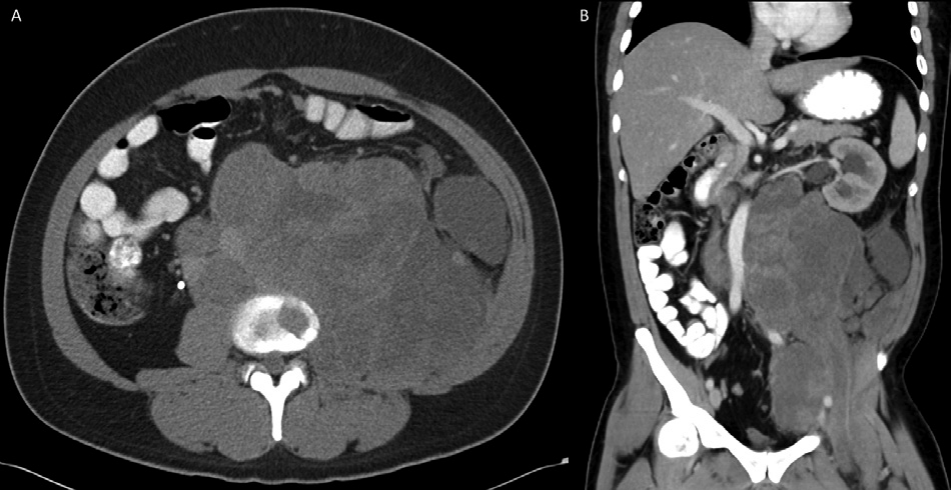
Axial (Fig. 1A) and coronal (Fig. 1B) computed tomography (CT) image of the abdomen 2 years following the initial diagnosis demonstrate a large heterogenous soft tissue mass arising from the left iliopsoas muscle. There was significant mass-effect on the abdominal viscera, with cranial displacement of the left kidney and right-ward deviation of the abdominal aorta.
